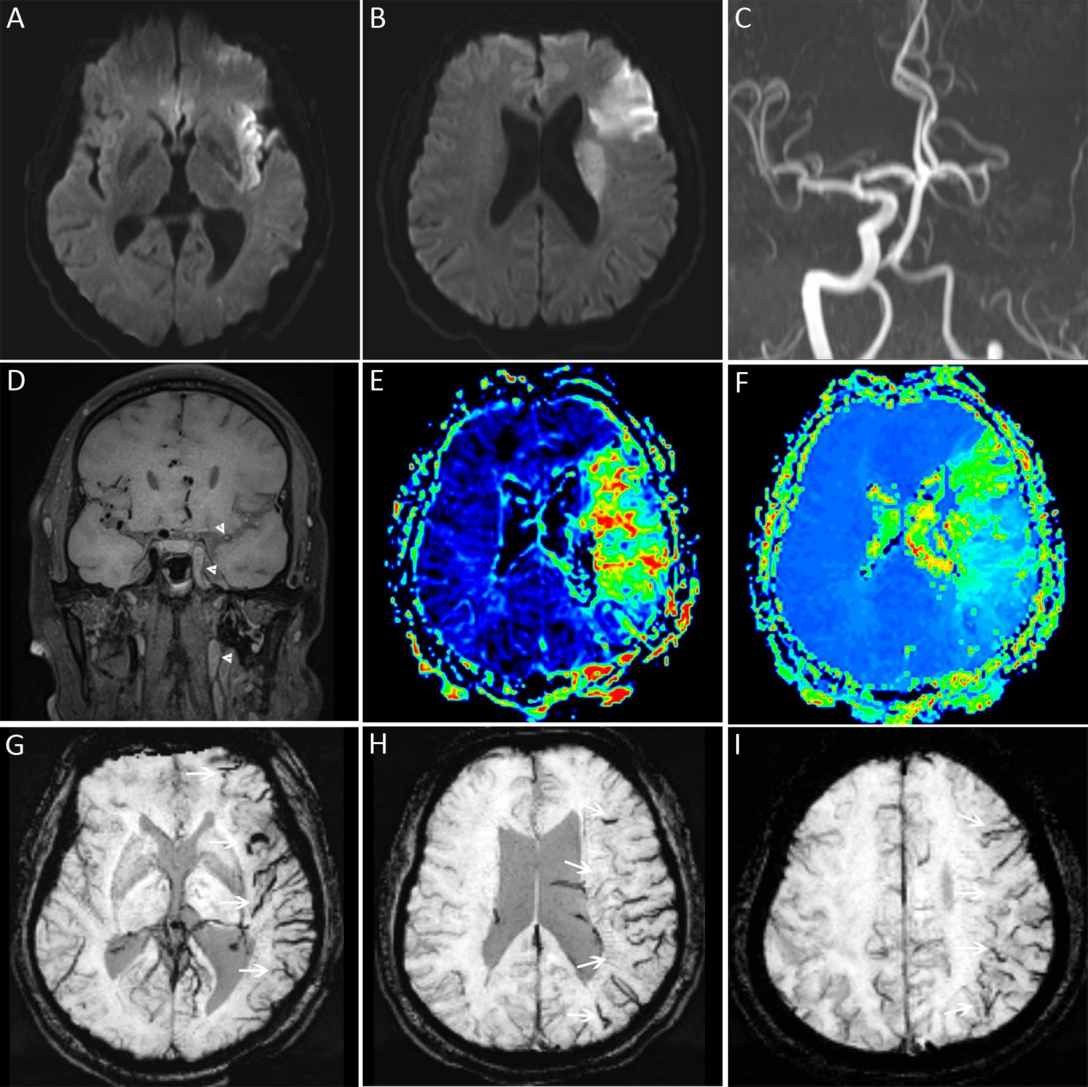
Prominent vessel sign was visible in a patient with anterior circulation stroke due to large vessel occlusion. DWI (A,B) revealed restricted diffusion in the left insular, frontal, and paraventricular areas. MRA (C) and 3D-SPACE (D) showed occlusion of the left internal carotid and middle cerebral arteries (white arrowheads). The MTT (E) and TTP (F) maps indicated hypoperfusion in the left middle cerebral artery territory. Asymmetric prominent hypointense vessels (white arrows), known as prominent vessel sign, appeared on SWI (G–I) in the affected regions.
